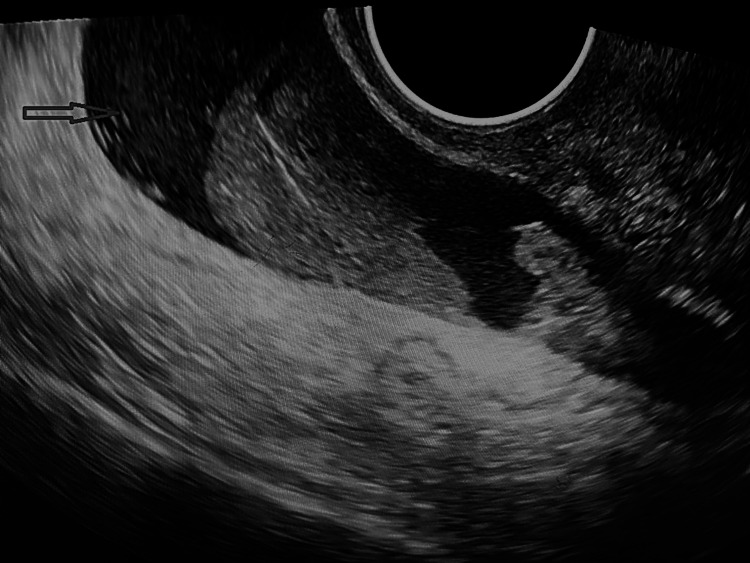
Gross hemoperitoneum evident on ultrasonography (arrow)
